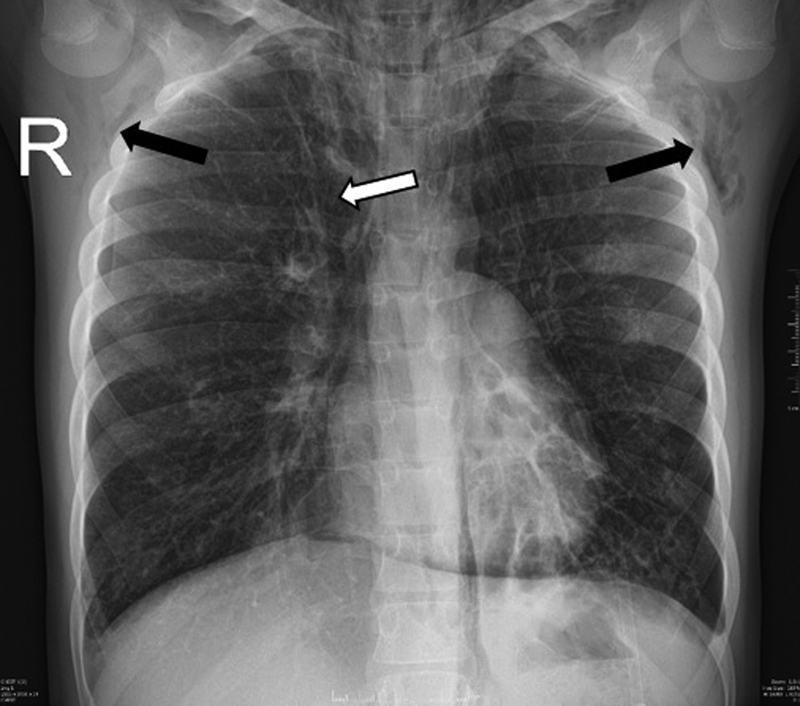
Chest X-ray, taken at the time of admission, also shows pneumomediastinum (white arrow); the subcutaneous emphysema is also visible (black arrows).
